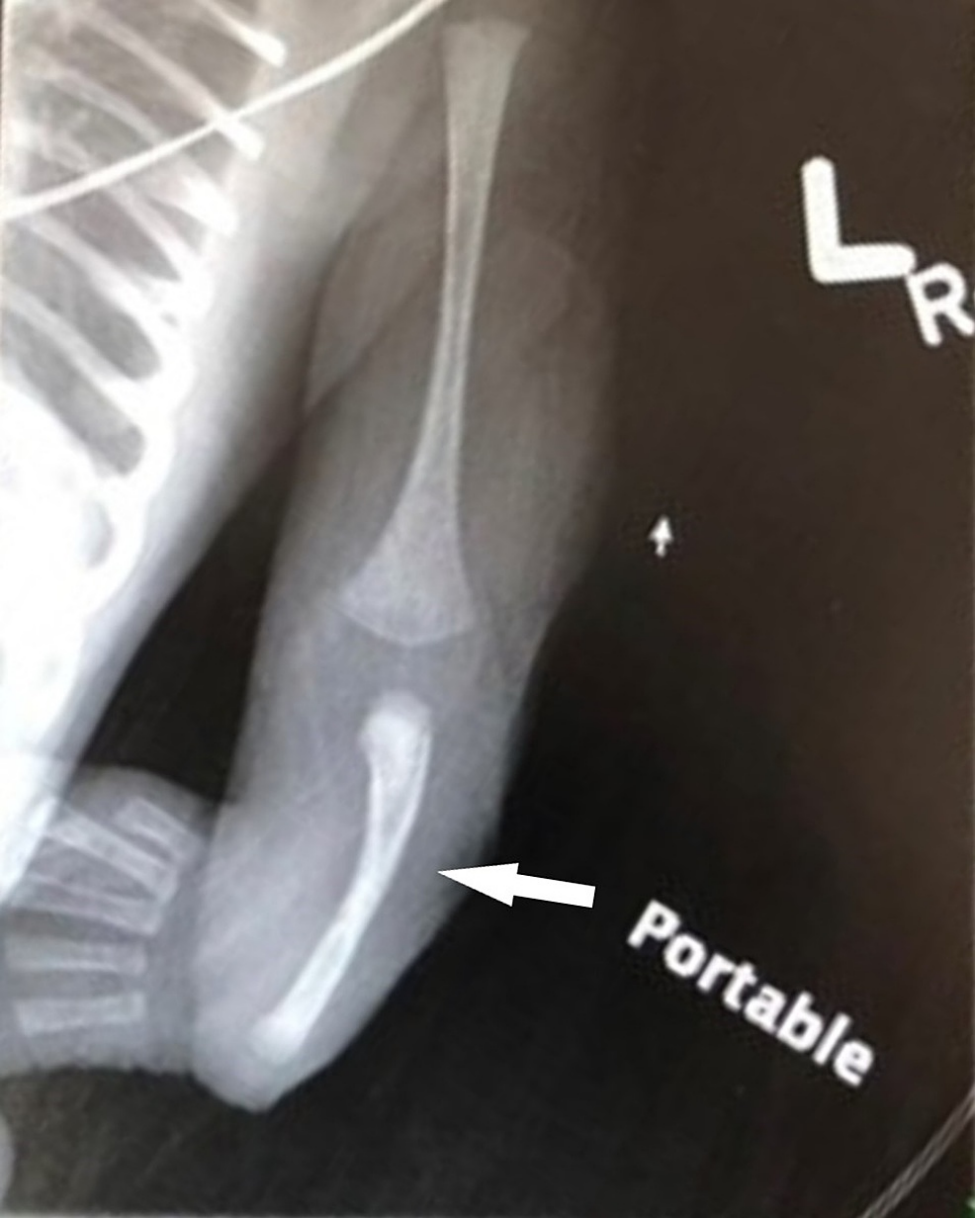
X-ray of the left arm demonstrating absent radius
